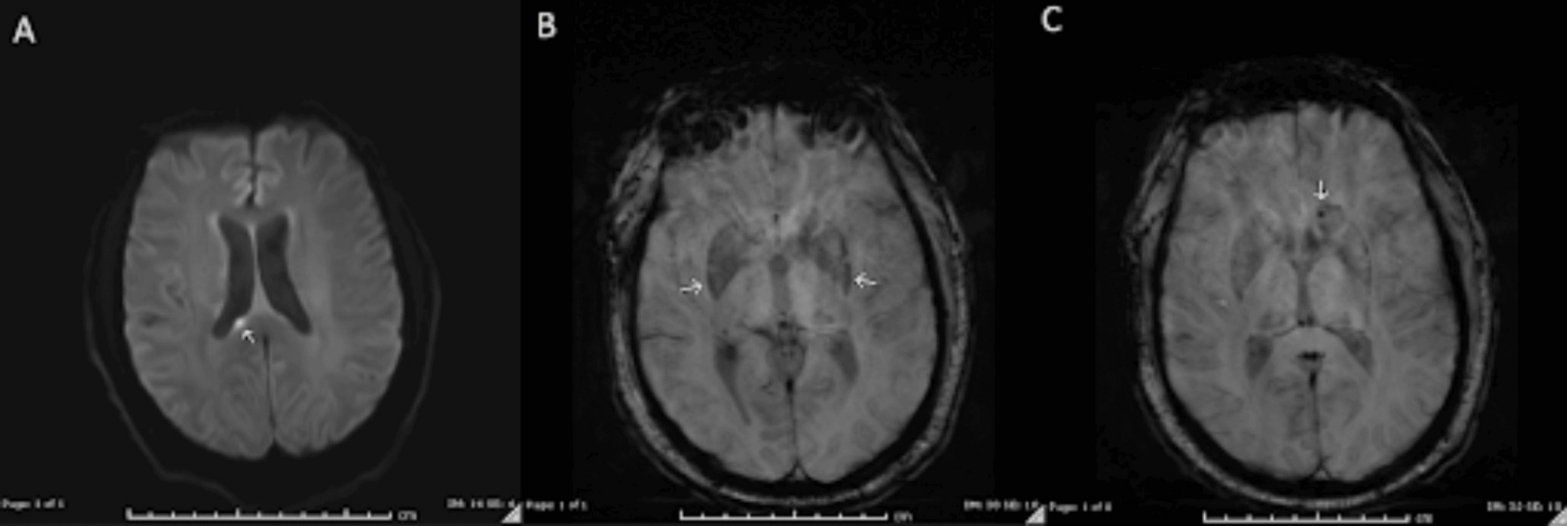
Repeat MRI 24 days after the original MRI (A) DWI: Area of diffusion restriction within the superior right splenium now measures 4 mm, unchanged accounting for differences in technique and measurement. (B-C) SWI: Increased conspicuity of a rim of susceptibility surrounding the left lentiform nucleus with interval development of more discrete foci of susceptibility within the head of the caudate nucleus on the left likely representing the interval development of microhemorrhages within the head of the caudate. MRI: magnetic resonance imaging; DWI: diffusion-weighted imaging; SWI: susceptibility-weighted imaging
